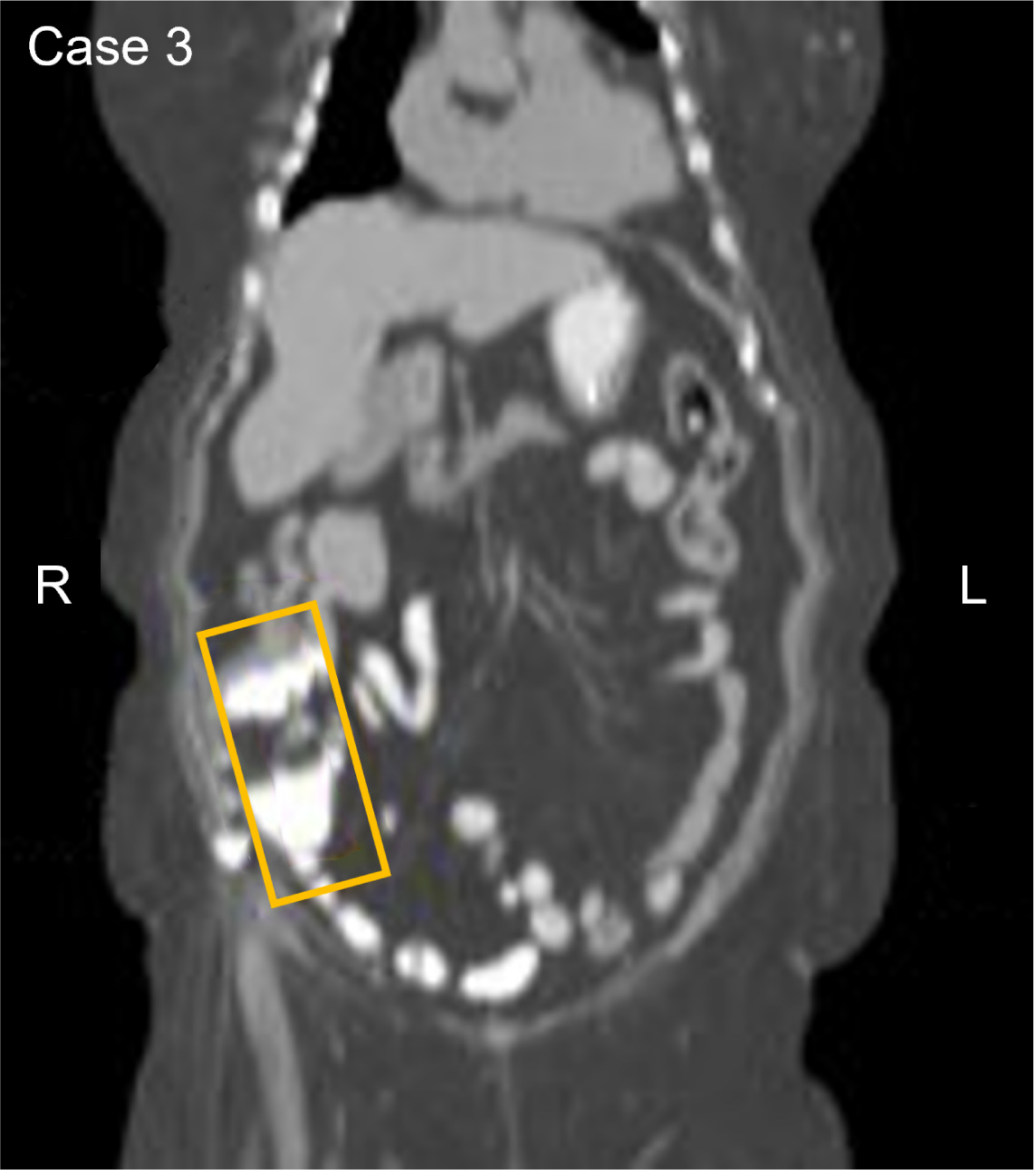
Participant three CT image. The area in the yellow box shows increased metabolic activity in the cecum and ascending colon with a filing defect suggesting a possible anomaly or irregularity in this region.
